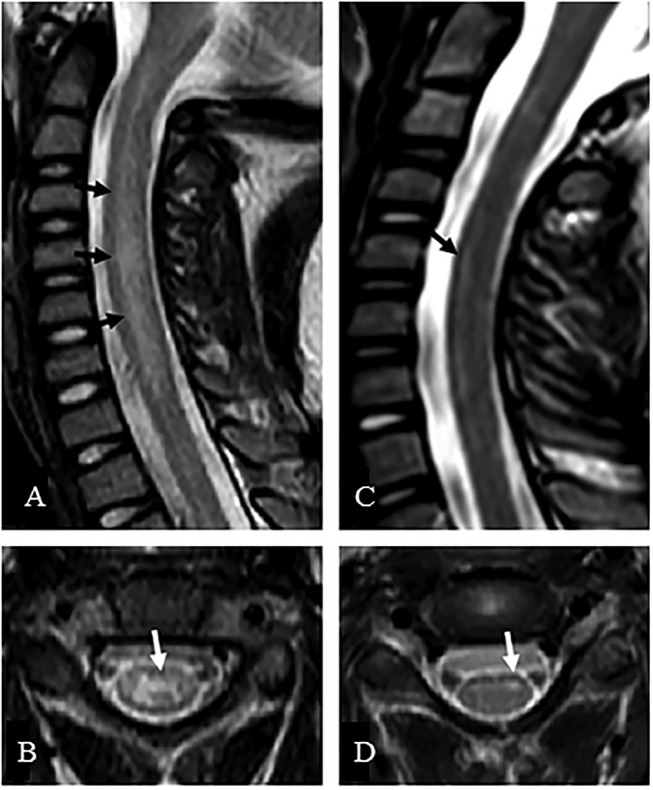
MRI of a suspected EV-D68 AFM patient. The MRI presents sagittal (A,C) and axial images (B,D) of the central nerves system. (A) Presents a case where the whole central gray matter was involved, producing a characteristic “H” pattern on axial image (B). (C) Presents a case where T2 hyperintensity was confined to the left anterior horn cells, which is demonstrated on the axial image (D). Taken from Maloney et al., 2015. Order License Id: 4382500446364.
